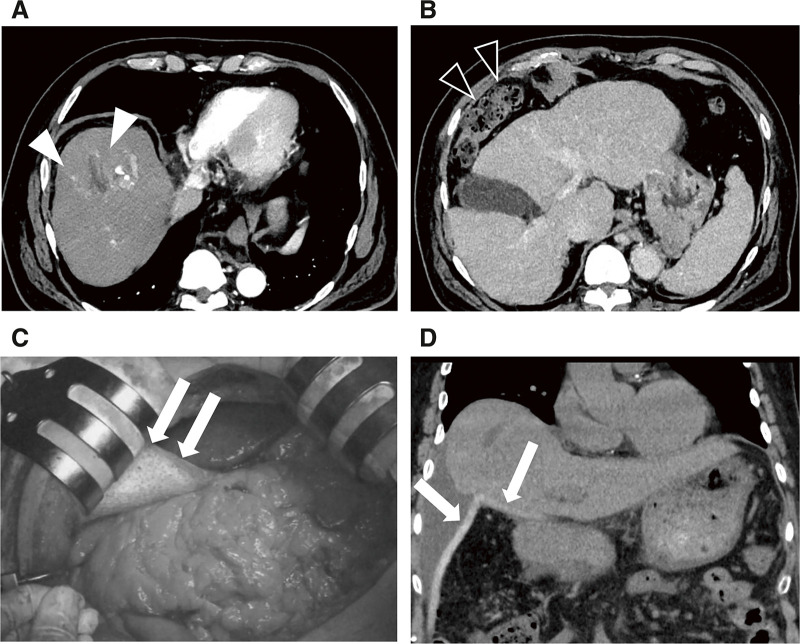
Abdominal computed tomography before and after the spacer placement surgery and intraoperative images. (A) Abdominal computed tomography revealing a 6 cm tumor located at segment 8 (white arrowheads). (B) The colon is located on the ventral side of the liver (black arrowheads). (C) A polyglycolic acid spacer (white arrows) is placed caudally to the liver. (D) Coronal slice computed tomography scan of the abdomen after the spacer placement surgery showing that the polyglycolic acid spacer and omentum prevented the colon from covering the ventral side of the liver (white arrows).
